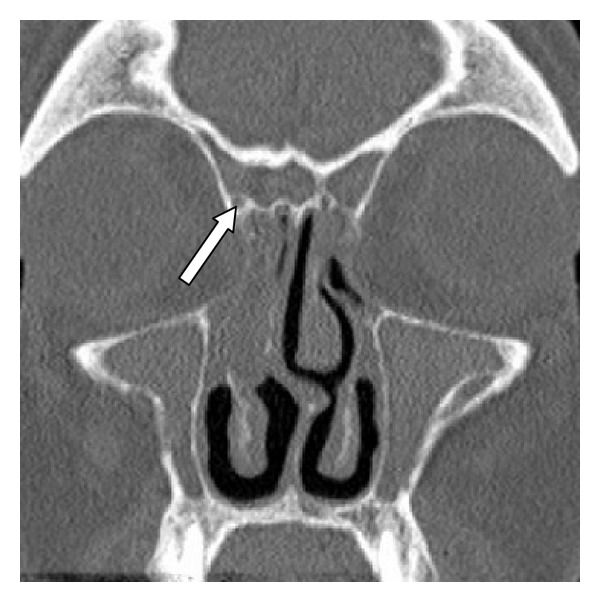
Small type III frontal cell (original figure). A coronal CT scan showing a small right-sided type III frontal cell (arrow) pneumatizing from the frontal recess into the frontal sinus. This cell, although too small to cause frontal sinus obstruction, is still included as a type III frontal cell.
